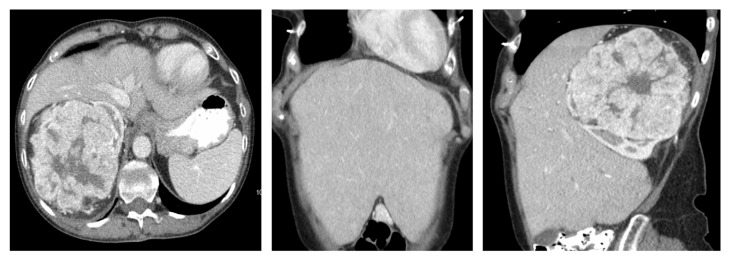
Abdominopelvic CT with IV contrast demonstrates a 14 cm enhancing mass in the right kidney. (a) Axial image shows there was significant enhancement of the solid components of the tumor with finger-like extensions of absent enhancement consistent with necrosis or inflammation. (b) Coronal image demonstrates the enlarged liver that filled all four abdominal quadrants. (c) In sagittal view, the liver is seen to be anteriorly displaced by the large renal mass and to extend down to the right iliac crest.
